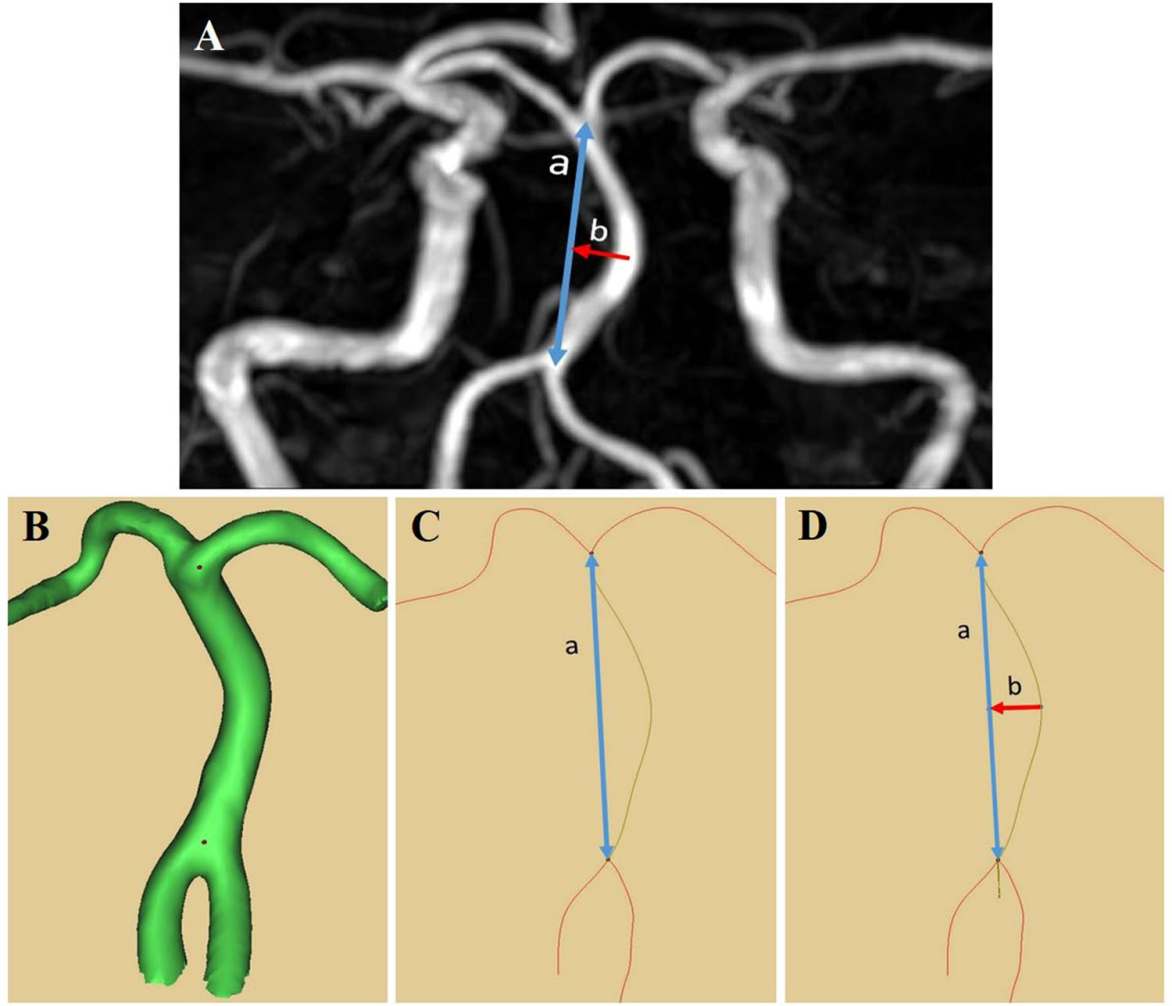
The geometry of the BA has been measured previously using magnetic resonance images (A), including the basilar artery length (a) and basilar artery bending length (b). Modification of method is used in order to improve the quantitative analysis using the MIMICS software (B-D).
